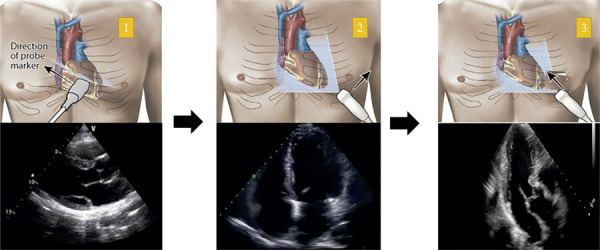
Echocardiographic views and probe positioning for the ValvUS protocol
